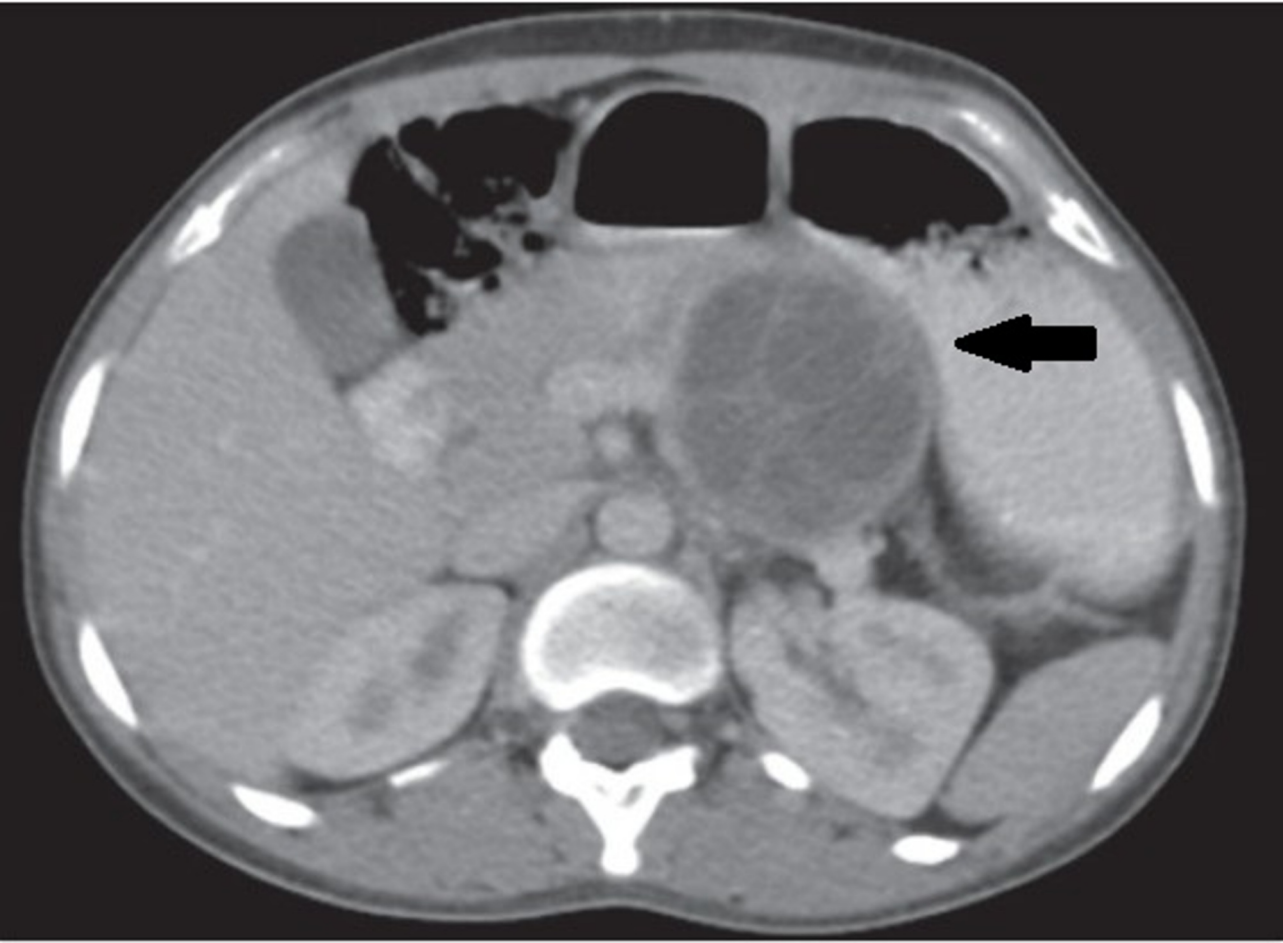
Hydatid cyst in pancreas on CT Axial CECT image shows a moderate–sized, well-defined, lobulated, thin, mildly enhancing walled intrapancreatic cystic lesion in the distal body and tail region of the pancreas (black arrow). The lesion shows multiple, internal, well-defined cystic areas/daughter cyst/septations, with no evidence of calcification. Mass effect is seen on the spleno-pancreatic axis, and the splenic vein is compressed and narrowed. CECT: contrast-enhanced computed tomography, CT: computed tomography
